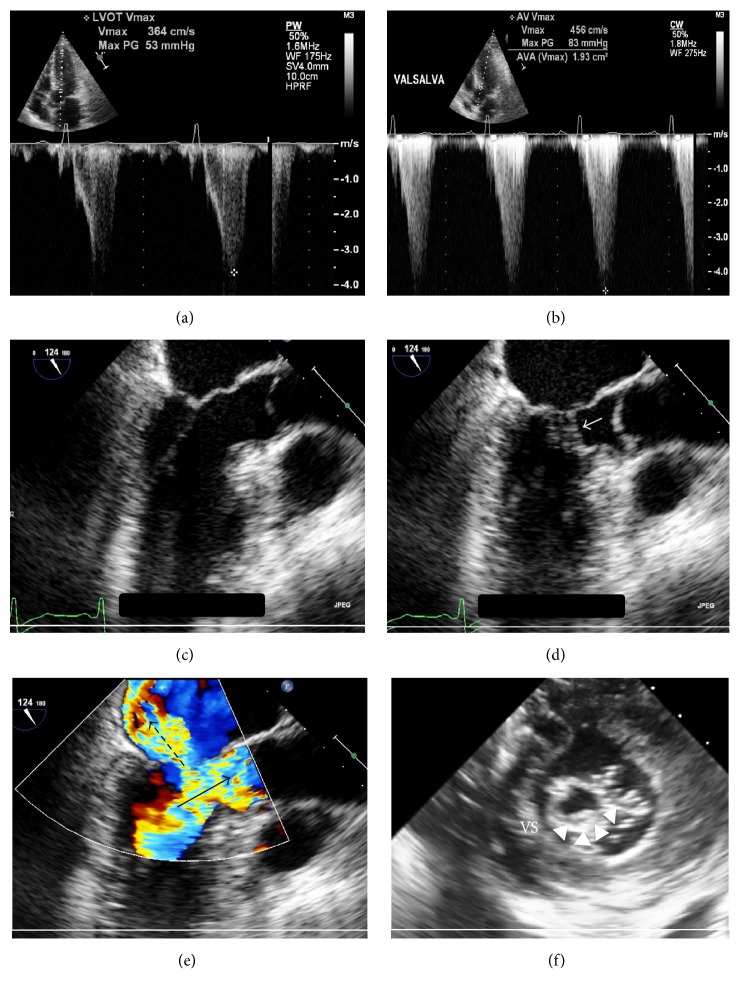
Transthoracic and transesophageal echocardiographic findings. (a) Pulse-wave Doppler at the LVOT showing a peak velocity of 3.94 m/s and gradient 62 mmHg. The waveform contour is dagger-shaped. (b) Strain-phase Valsalva results in an increase in peak velocity to 4.56 m/s with gradient 83 mmHg. (c) Apical view at end-diastole showing no significant LVOT narrowing. The septal wall shows moderately increased thickness measured at 1.4 cm. (d) Apical view during systolic phase showing mitral-septal contact (white arrow) consistent with SAM. (e) Color Doppler showing severe, posteriorly directed mitral regurgitation (dashed arrow) into a moderately enlarged left atrial cavity and significant turbulence across the LVOT (solid arrow). (f) Transgastric short-axis view at the level of the mitral valve during systolic phase, showing a large echodensity attached to lateral scallop of the anterior mitral leaflet (arrowheads).
